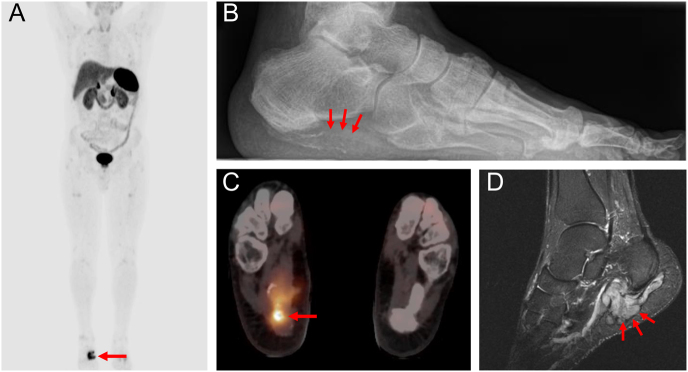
Multimodal imaging approach for localization of a PMT in the foot. Initial plain radiograph (B) revealed a subtle, partially calcified lesion within the soft tissue close to the calcaneus (red arrows). Functional imaging using ^68^Ga-DOTATATE PET/CT demonstrated intense radiotracer uptake at the lesion site (C), and whole-body PET localized the tumor to the right foot with high sensitivity (A). Anatomical imaging with MRI (D) showed a T2w hyperintense, contrast-enhancing, well-demarcated lesion consistent with a PMT.
